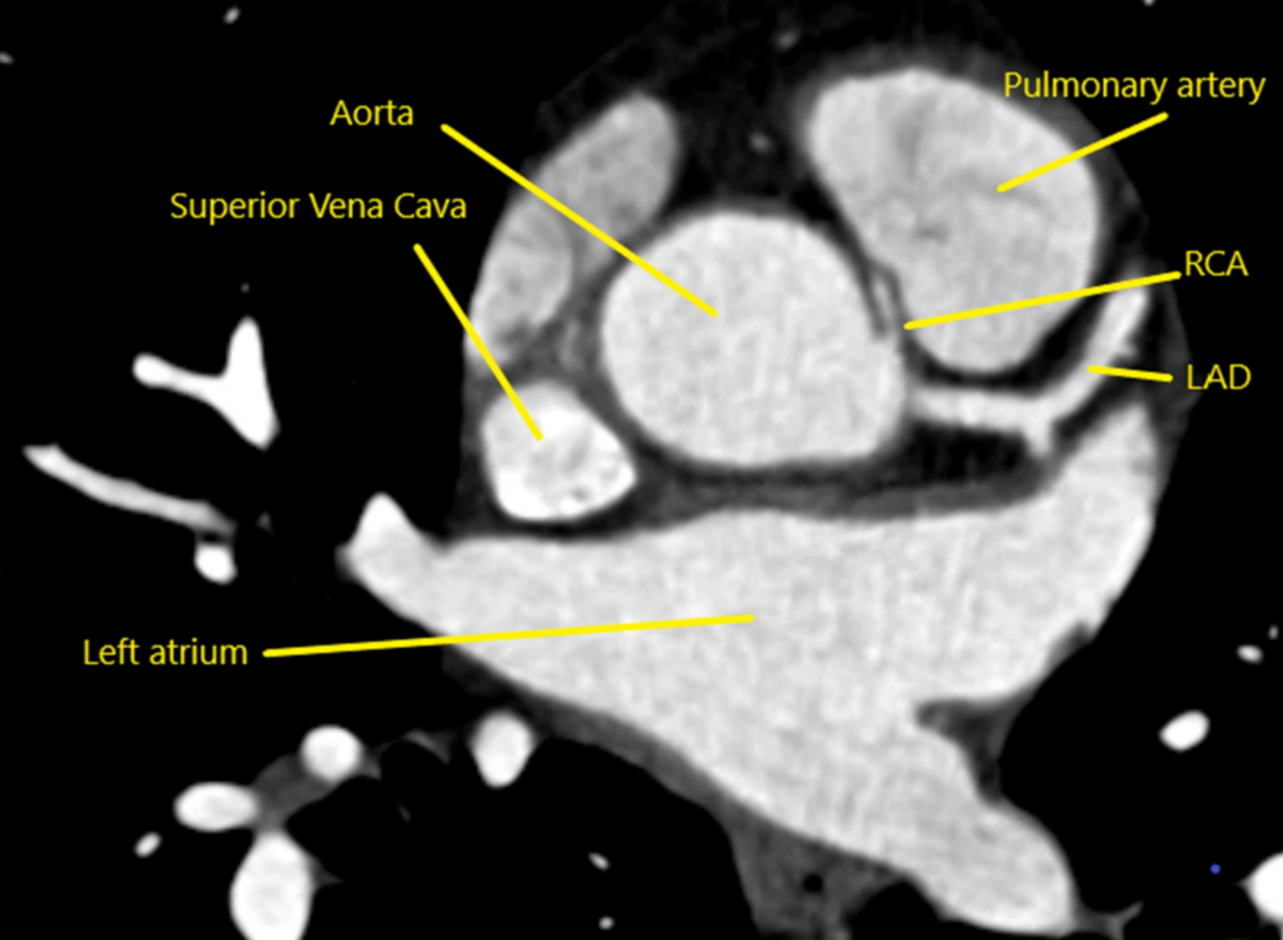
Computed tomography angiography, anomalous origin Anomalous origin of the right coronary artery from the left sinus of Valsalva. LAD=left anterior descending artery; RCA=right coronary artery.
